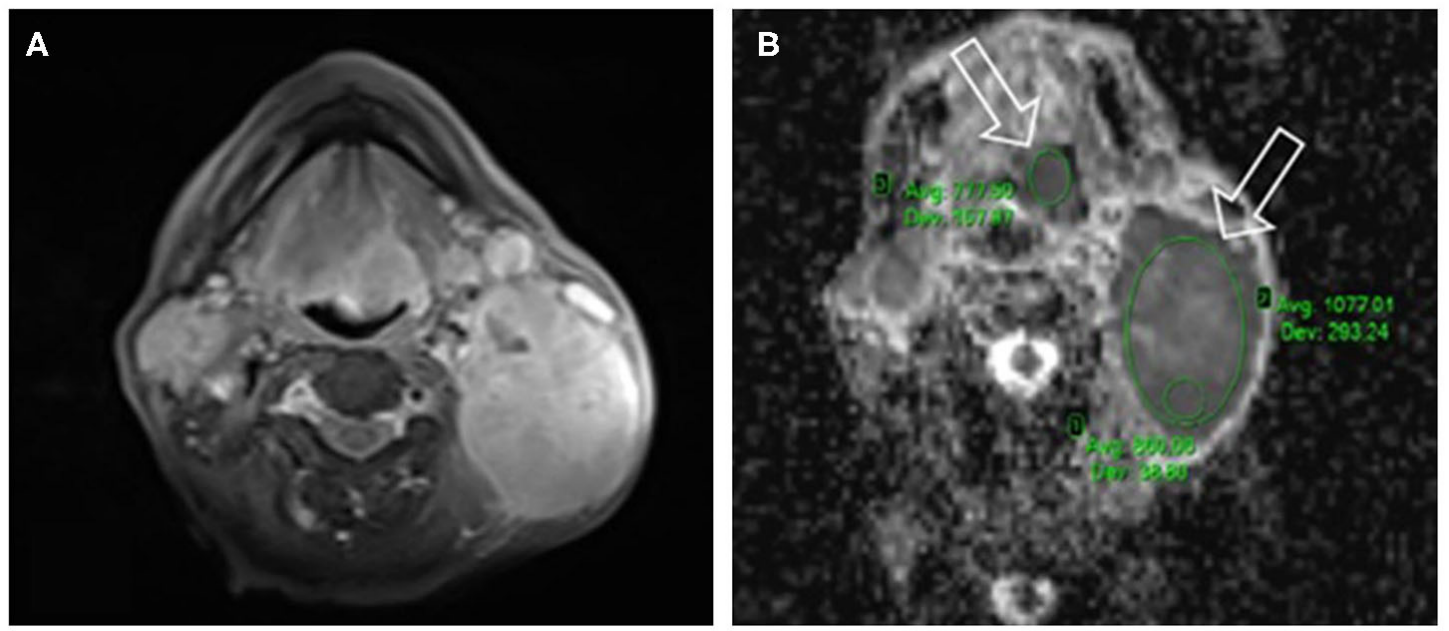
Axial Dixon fat suppressing (FS) T1-weighted turbo spin echo (TSE) sequence (A) used as an anatomical map. On the corresponding apparent diffusion coefficient (ADC)-map (B) the area of low signal intensity, epiglottic primary tumor's (left white arrow) mean ADC (ADC_mean_: 777.5 + -42.2 10^−6^ s/mm^2^) delineated (with left green ellipse), and the metastasis of largest lymph node (right white arrow) on the left side of the neck.
